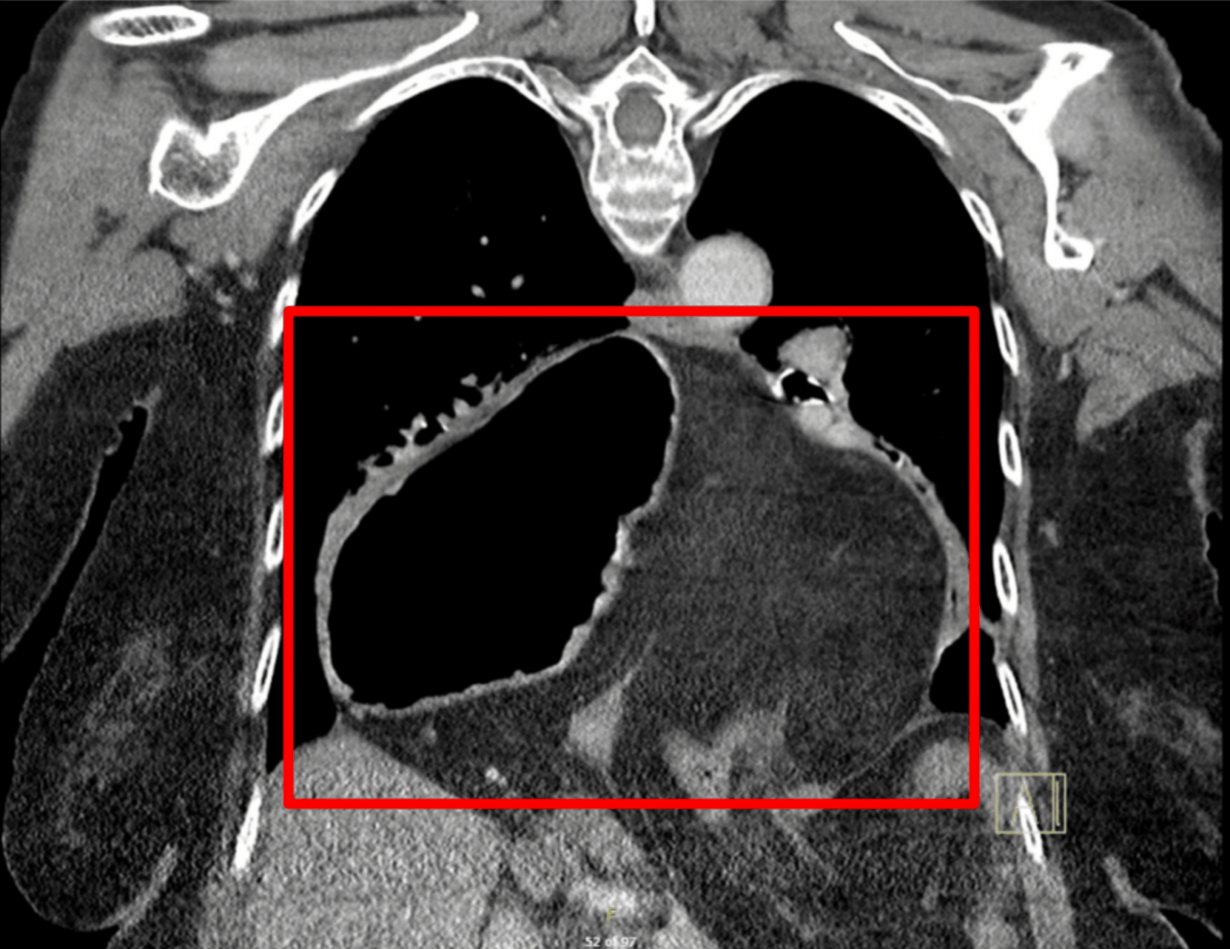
Anterior-posterior chest CT scan with contrast demonstrating a giant hiatal hernia. The CT image reveals a massive hiatal hernia, outlined by the red box, measuring at least 18 cm in transverse diameter. The hernia contents, visible within the thoracic cavity, include the entire stomach, proximal duodenum, and a segment of the transverse colon. This extensive herniation significantly alters the normal anatomical relationships within the chest and upper abdomen, contributing to the complex clinical presentation in this elderly patient.
